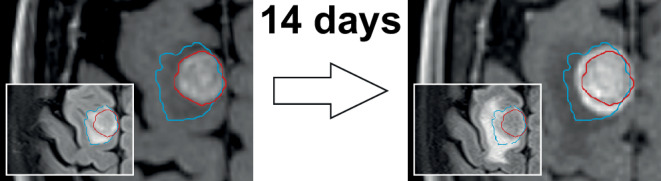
Importance of minimizing the time interval between imaging and SRT. Considerable tumor growth in a patient with brain metastasis in only 14 days (3D T1w). *Inset*: Increase in perifocal edema (T2-FLAIR) causing a shift in tumor position
